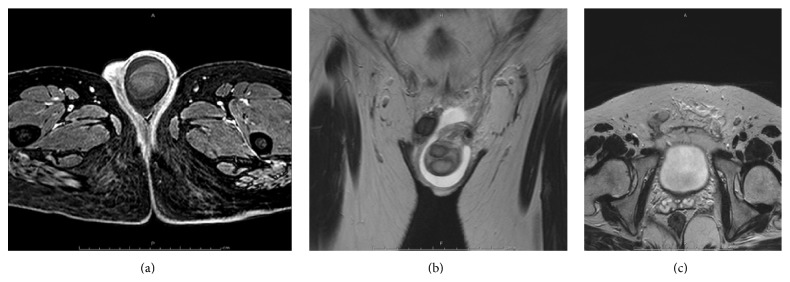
Contrast-enhanced magnetic resonance imaging. (a) No enhancement and inhomogeneous signal of the left testis. (b) Torsion of the left testis. (c) Cryptorchidism of the right testis.
